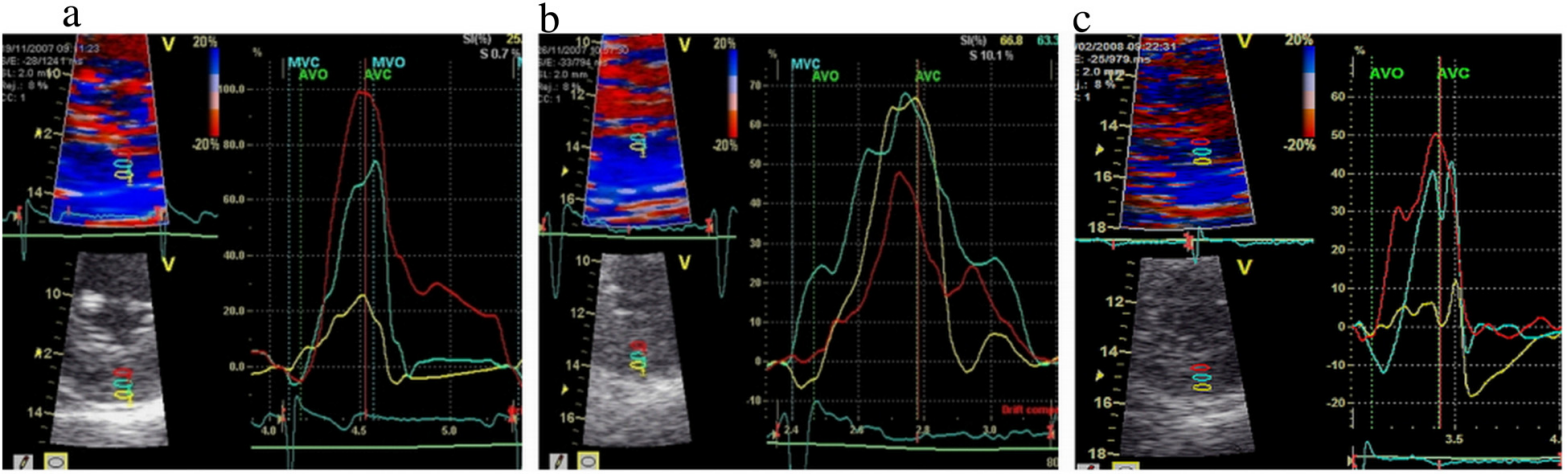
Measurement of peak systolic radial strain in three layers in the left ventricular inferior wall of: 1a) one normotensive patient with non-severe aortic stenosis; 1b) one normotensive patient with severe aortic stenosis; 1c) one hypertensive patient with severe aortic stenosis. Each panel: Top left – Colour TDI image in parasternal short-axis view of the inferior left ventricular wall. Bottom left – B-mode image with 3 regions of interest placed in three layers (subendocardium, mid-myocardium, subepicardium) in the left ventricular inferior wall. Right panel: corresponding peak systolic radial strain curves for the three regions of interest: red curve for subendocardium, blue curve for mid-myocardium, and yellow curve for subepicardium.
